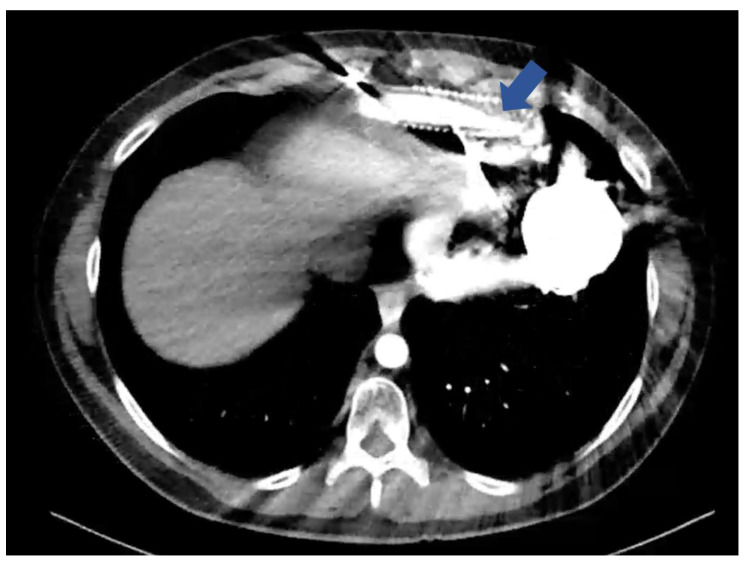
Contrast-enhanced CT-angiography of a patient with eOGO (blue arrow). CT, computed tomography; eOGO, external compression of the outflow graft causing obstruction.
